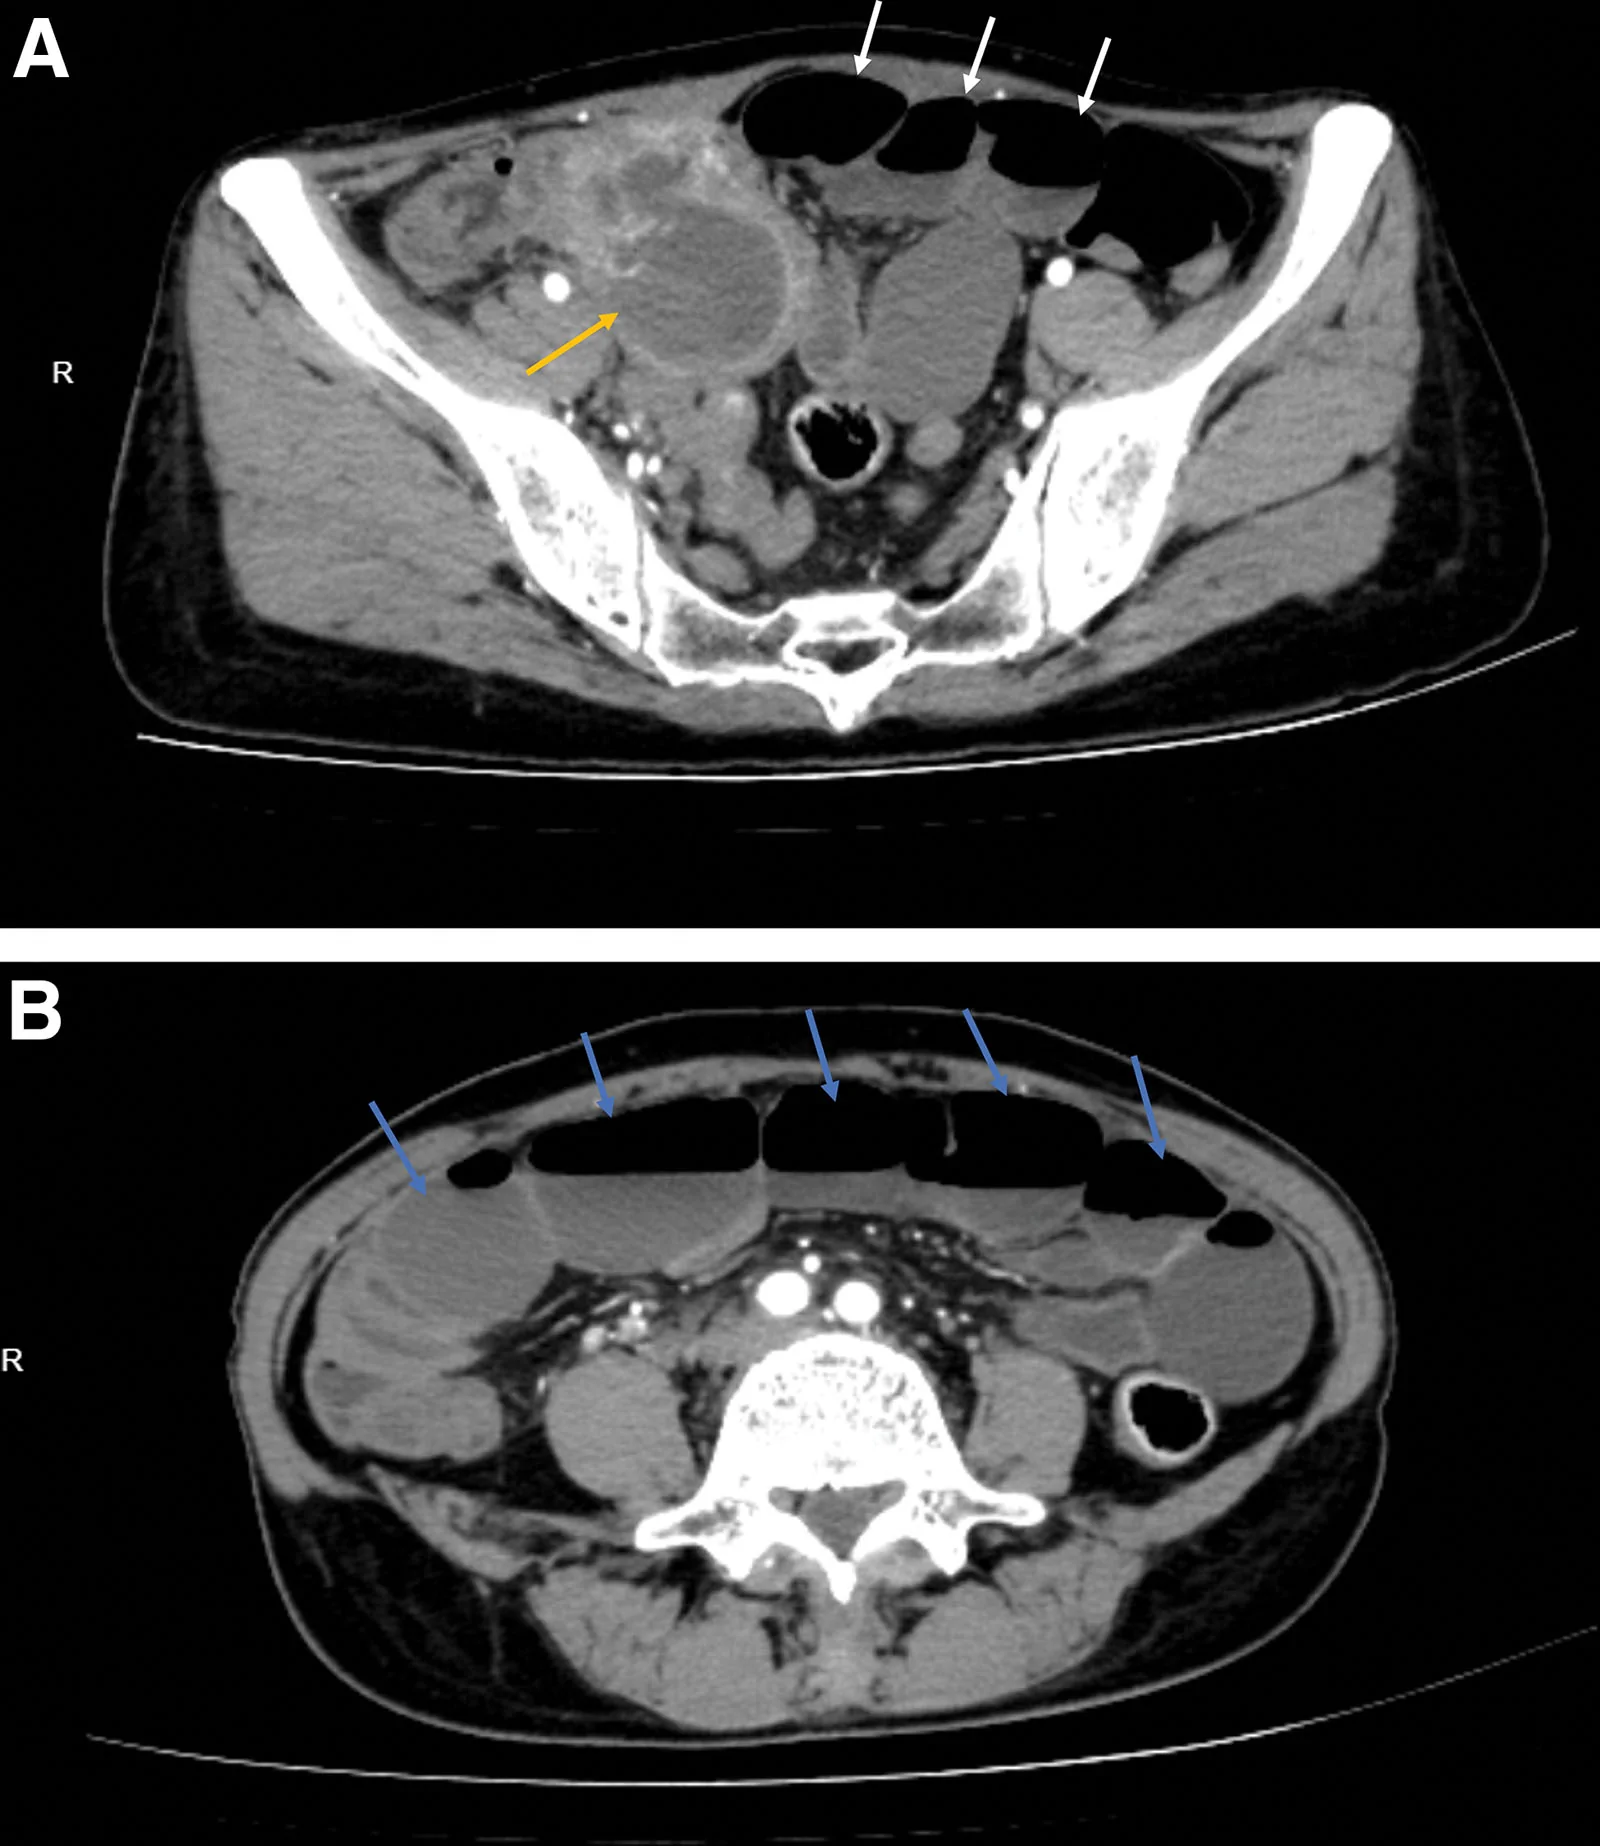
(A) Contrast-enhanced CT image showing a well-defined appendiceal abscess (yellow arrow) causing upstream small-bowel dilation (white arrow). (B) The small-bowel dilation did not show significant relief (blue arrow). CT = computed tomography.
